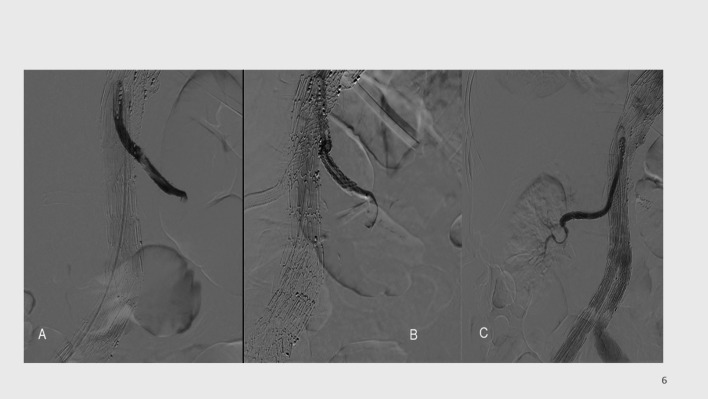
A 76-year-old patient with a thoracoabdominal aortic aneurysm was treated with T-branch off-the-shelf stent graft. Baseline eGFR was 89 mL/min. Three months after BEVAR, the patient was admitted to our hospital four days after the onset of anuria. Bilateral stent graft thrombosis was confirmed on CT, and recanalisation of both renal arteries was attempted. The left renal artery could not be recanalised, and there was no flow in the segmental renal arteries (A, B). The right renal artery was successfully recanalised, with restoration of flow in the main renal artery and two segmental arteries; however (C), there was no clinical improvement, and the patient remains on permanent dialysis
